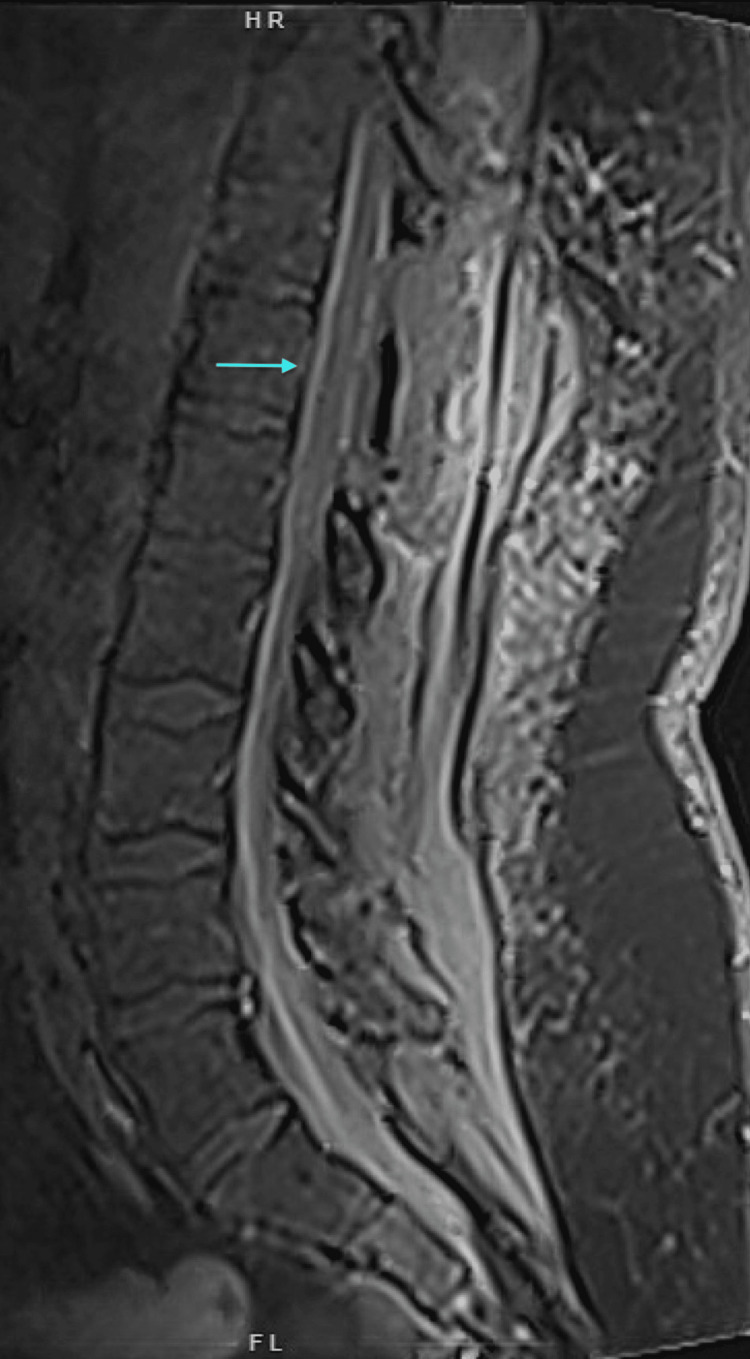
Postoperative Magnetic Resonance Imaging of Spinal Cord Evacuation of the hematoma and postoperative changes after laminectomy.
